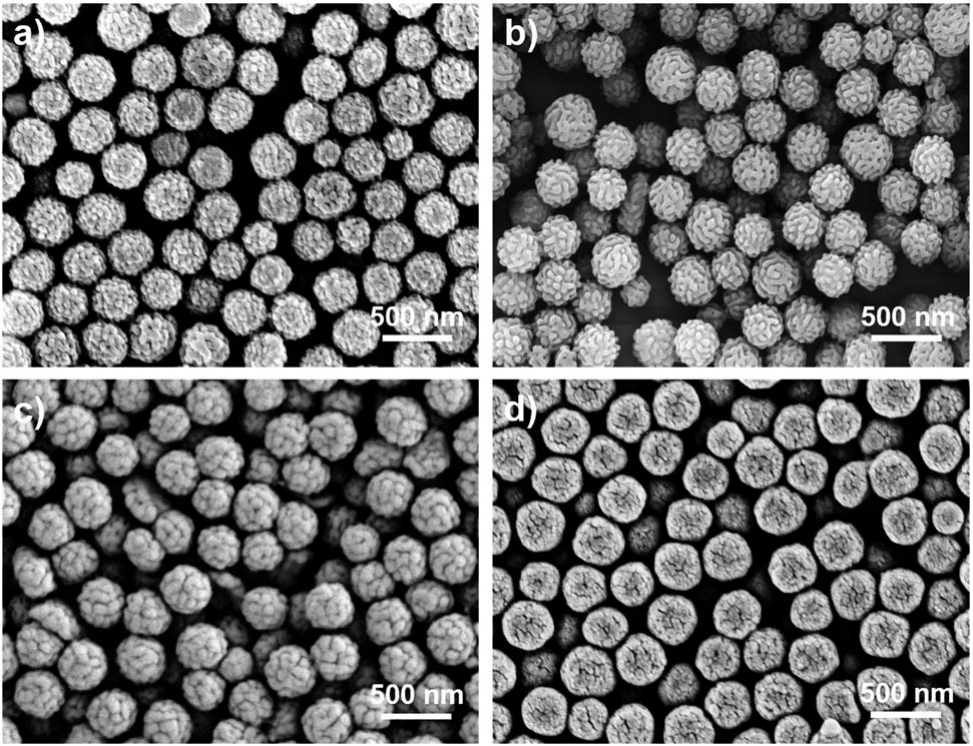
SEM images of RB particles synthesized at [PFS]/[PDMA_54_-CTA] = (a) 100/1, (b) 200/1, (c) 300/1, and (d) 500/1.
